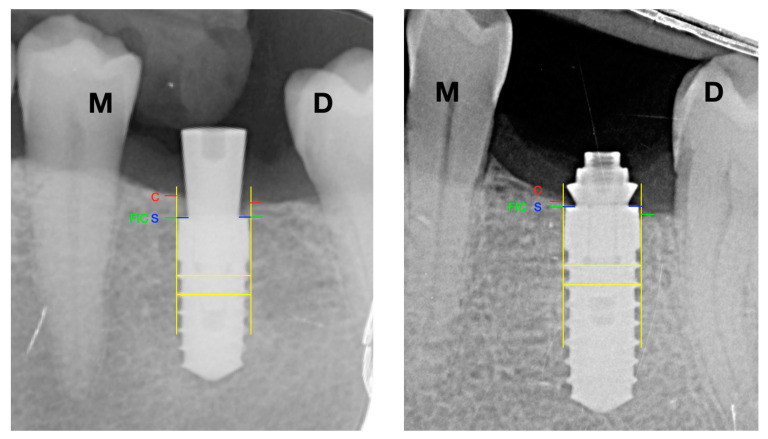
Radiographic analysis. S: implant shoulder; C: bone crest; FIC: first bone to implant contact; M: mesial; D: distal; SC distance: marginal bone level at the crest; S-FIC distance: marginal bone level at the implant.
